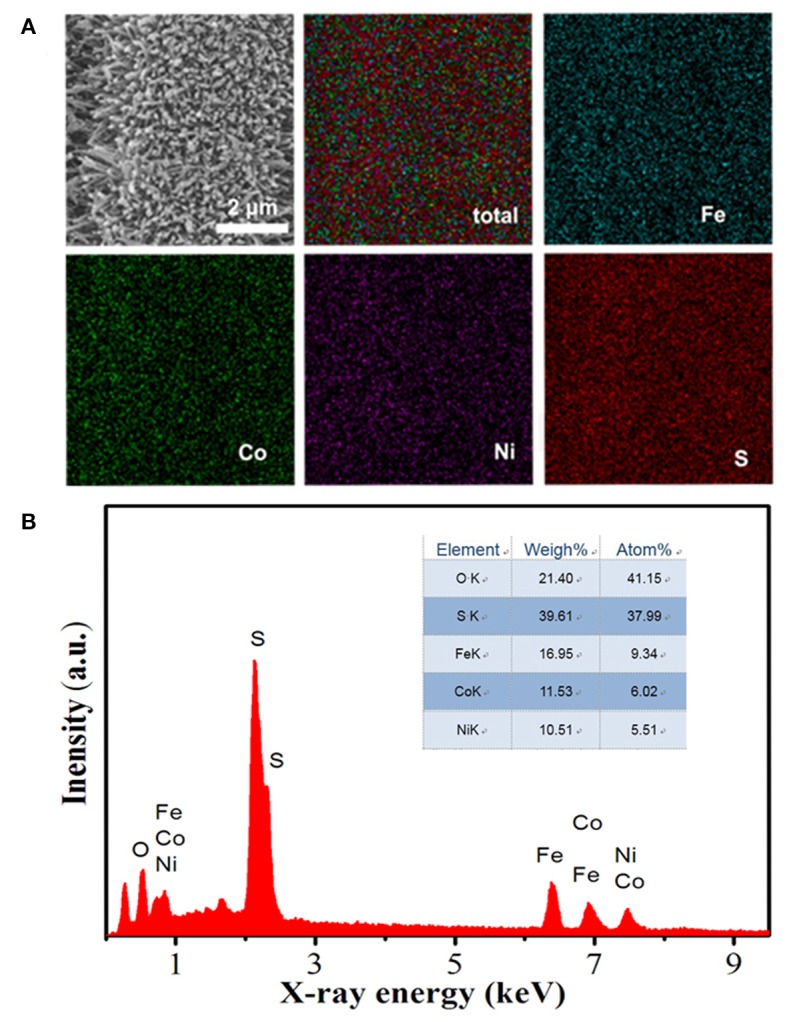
(A) Energy-dispersive X-ray (EDX) mapping images of FeCoNiS-FeOx. (B) EDX element distribution pattern.
